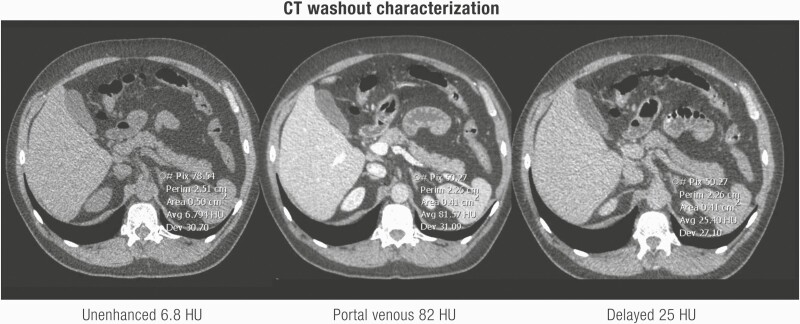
Characterization of AI by CT. Axial images obtained pre and post-IV contrast at the portal-venous (60-70 seconds) and delayed (10-15 minutes) phases post injection. Calculation of adrenal lesion attenuation value showing benign pattern washout in a lipid-rich left adrenal adenoma.
